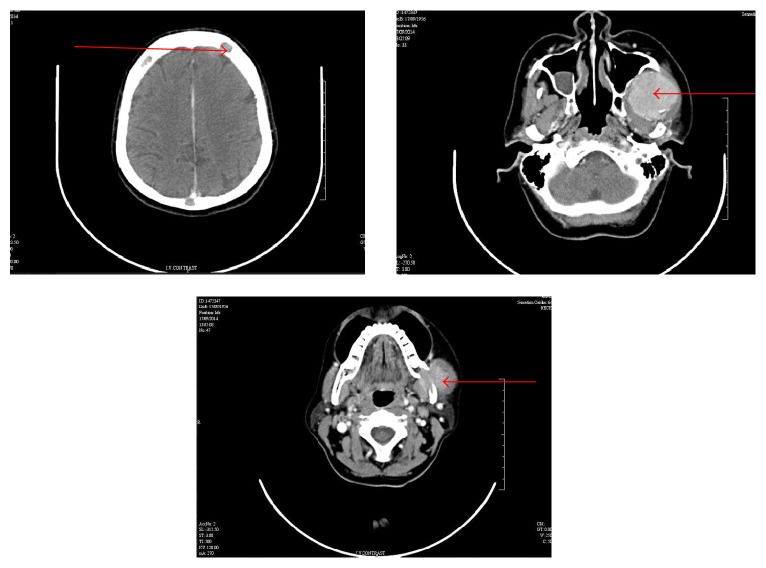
Axial CT section: Lytic lesion in left frontal bone and an expansile lytic and destructive lesion in the left ramus of the mandible involving the left infratemporal fossa and masseteric space and indenting the lateral wall of the left maxillary sinus.
